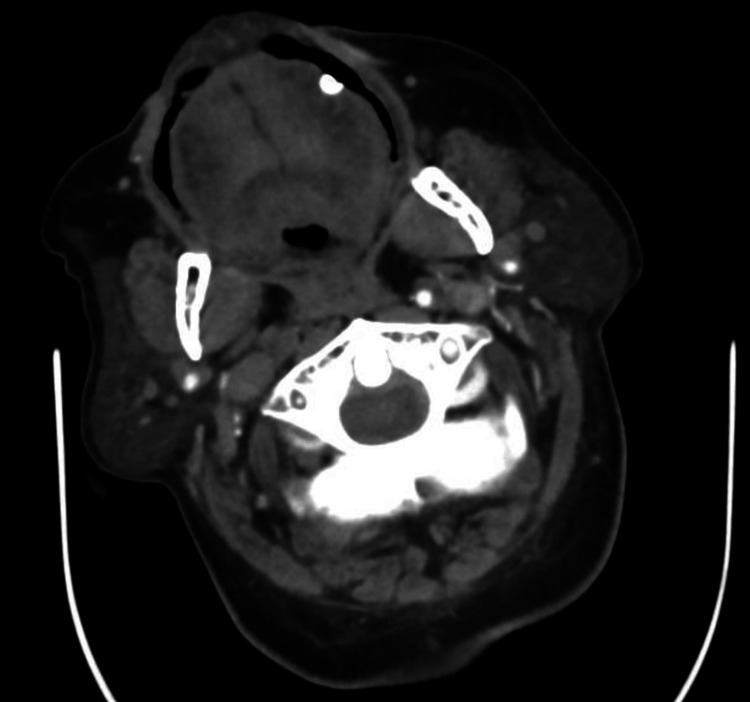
CT angiogram (axial view) showing the right ICA with non-opacification from its proximal portion to the clinoid segment intracranially. ICA, internal carotid artery
